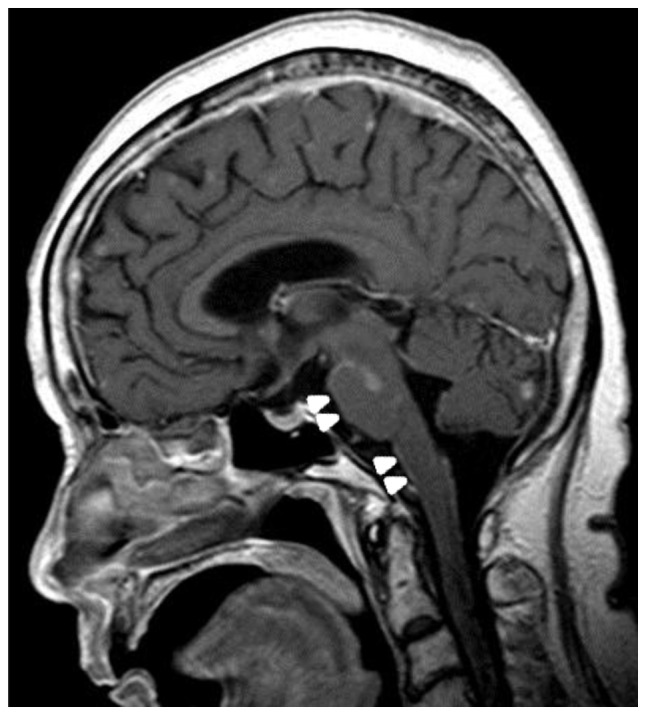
Gadlinium-enhanced T1WI magnetic resonance imaging on the 33rd day of hospitalization. The gadolinium-enhanced meninges in front of pons and medulla are shown (arrowheads).
